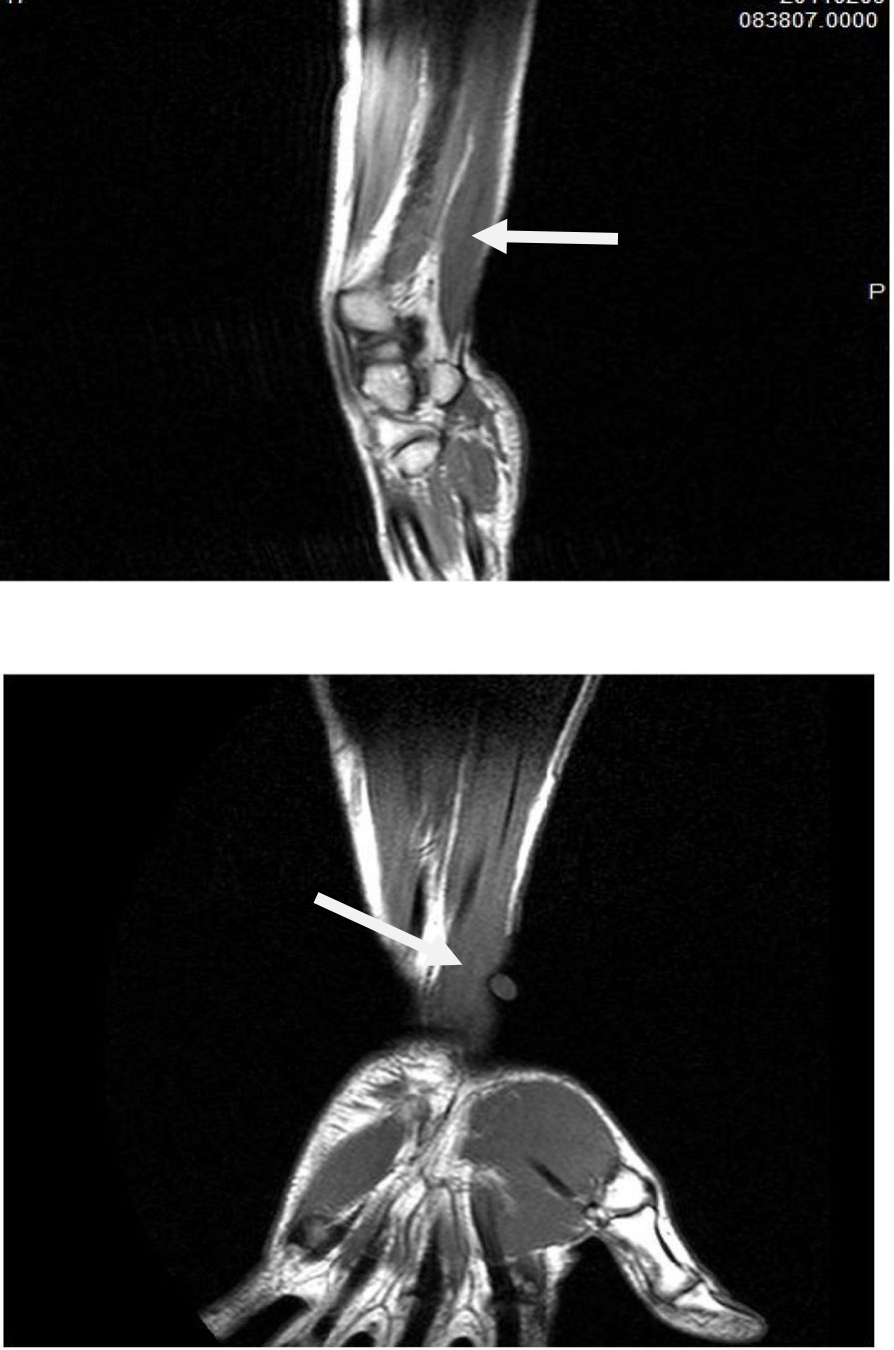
T1-weighted (a) sagittal and (b) coronal magnetic resonance images of the right distal forearm showing the mass isointense with the muscles of the forearm (the *arrow* indicates the hypertrophied muscle)
